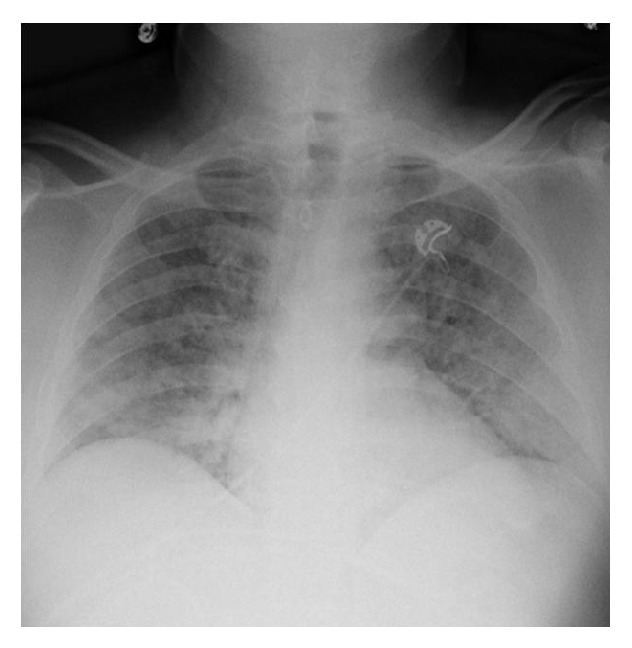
Chest X-ray from hospital day 3 showing worsening bibasilar opacities.
